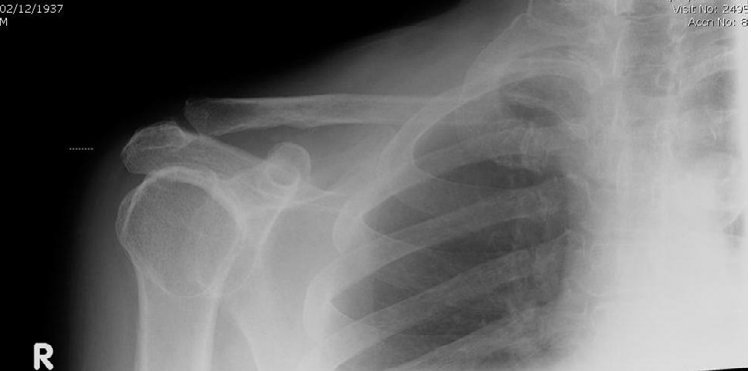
Plain radiograph of right clavicle. The radiograph demonstrates a pathological fracture of the medial aspect of the right clavicle.
